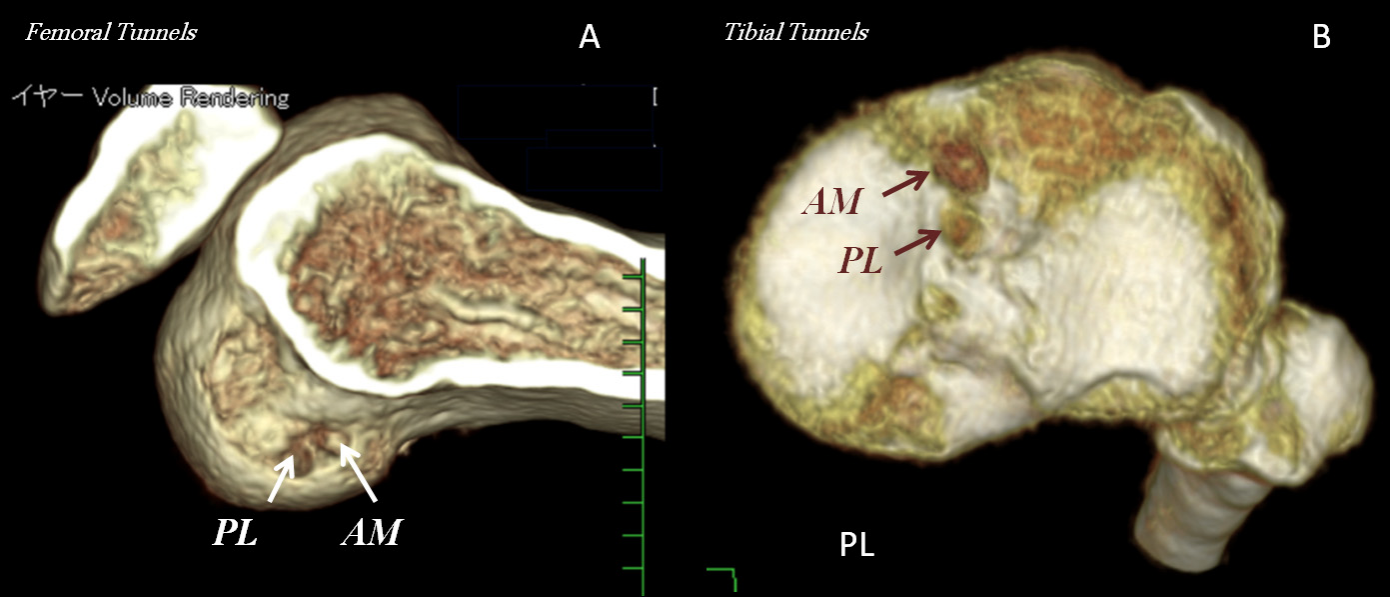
Post-operative 3D CT images of AM and PL tunnels (A: femoral side, B: tibial side). These 3D CT images prove that our technique allows for anatomical double bundle reconstruction without the need for the image intensifier for intra-operative tunnel localization.
